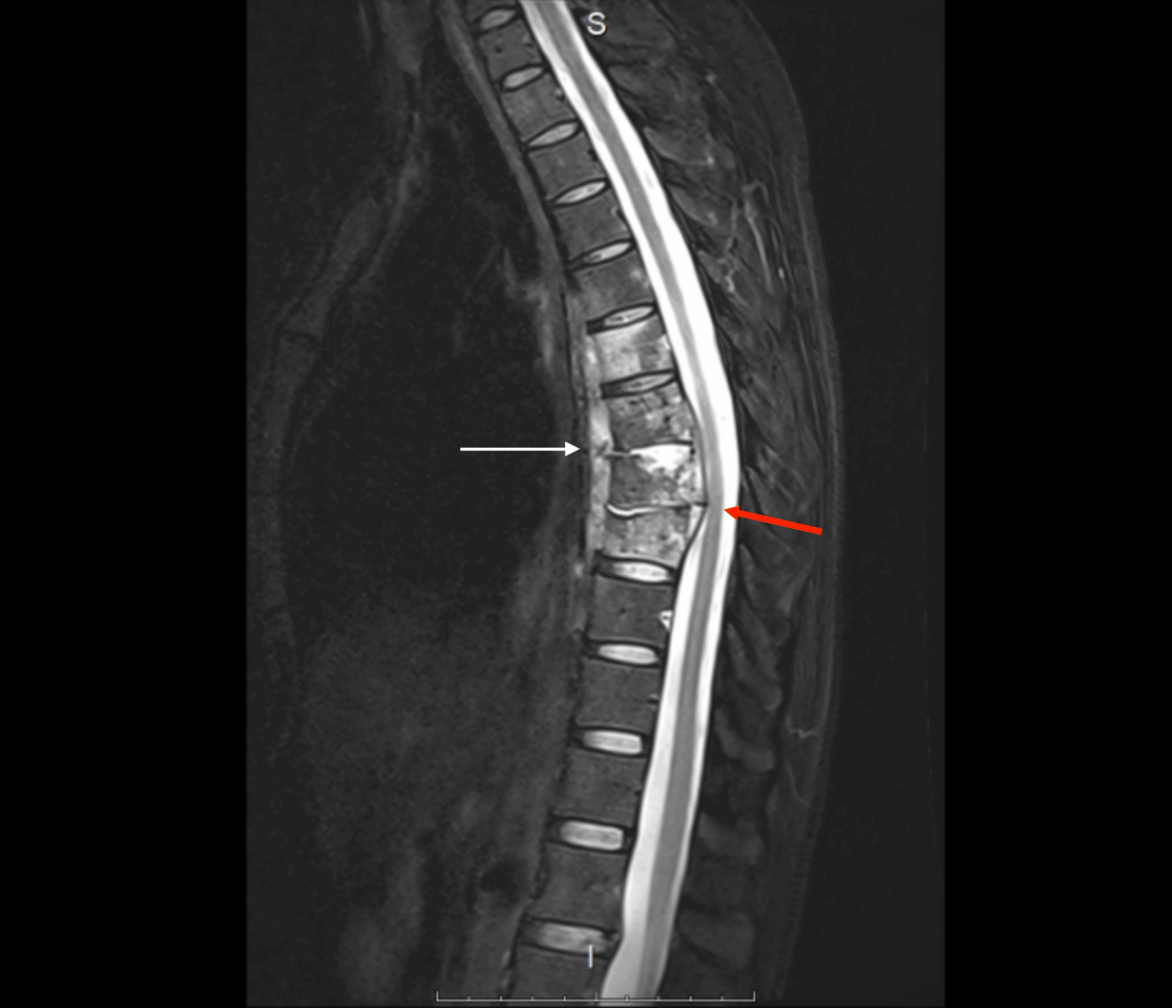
Sagittal MRI of the thoracic spine showing the prevertebral cold abscess. The white arrow indicates the abscess, and the red arrow indicates narrowing of the anterior subarachnoid space. MRI: magnetic resonance imaging
